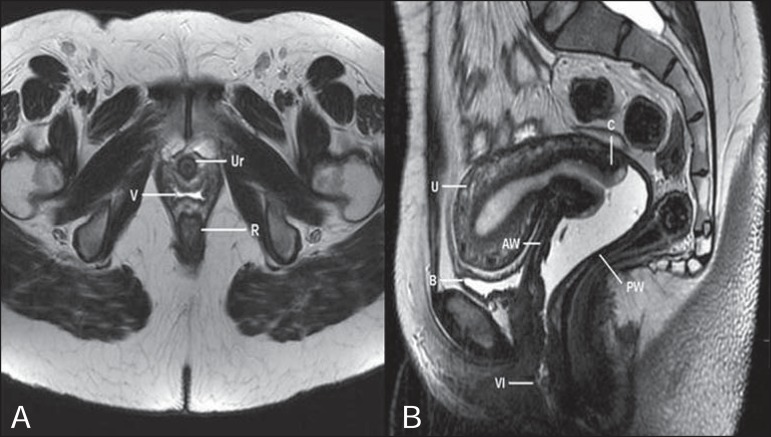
Normal female pelvis. Axial (A) and sagittal (B) MRI
